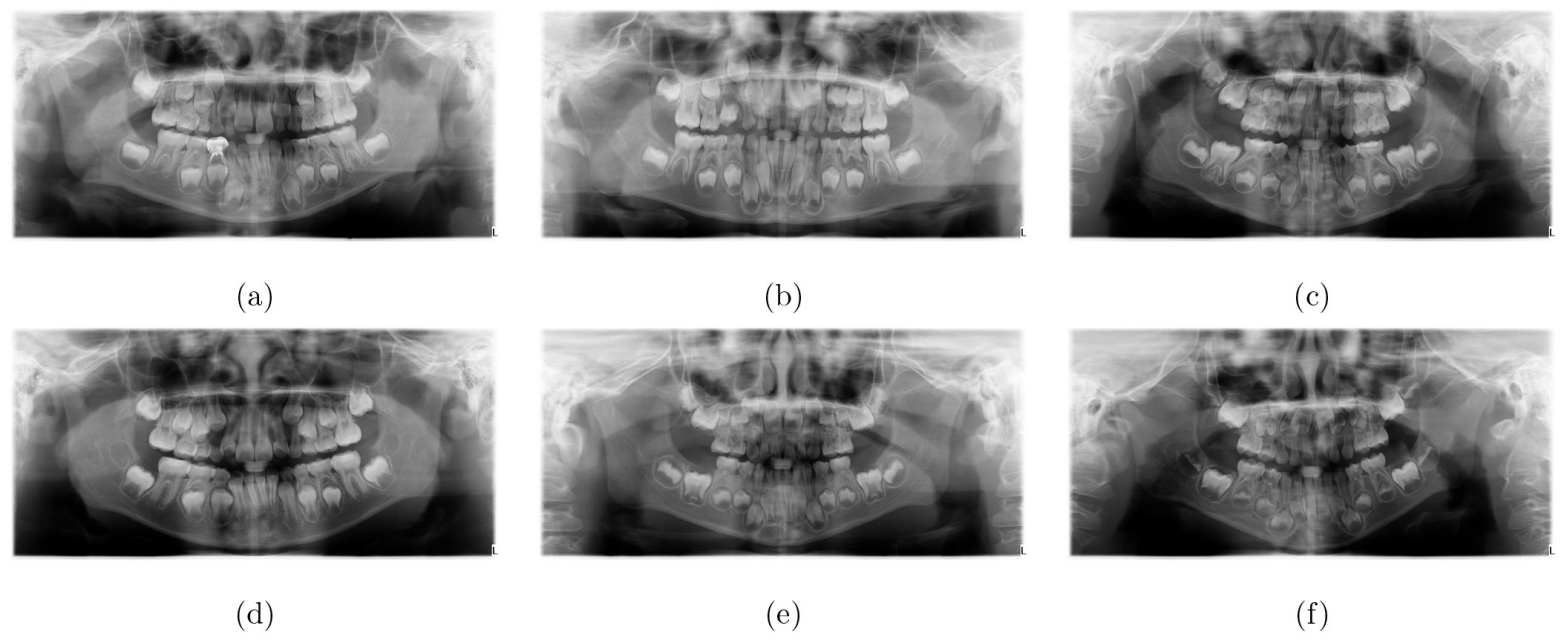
Reduced-resolution panoramic radiographs of pediatric dental diseases: caries or class 1 (a–c), and periapical infections or class 2 (d–f).
